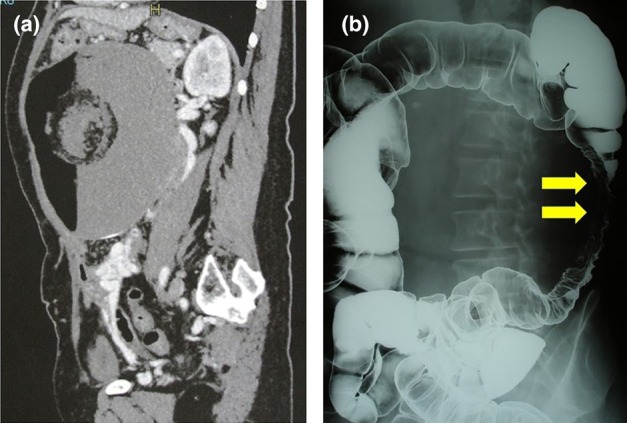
Computed tomography and barium enema of the abdomen. (a) A spherical tumor containing liquid was observed anterior to the left kidney. (b) The descending colon was narrow, and its wall was irregularly transformed by the tumor.
